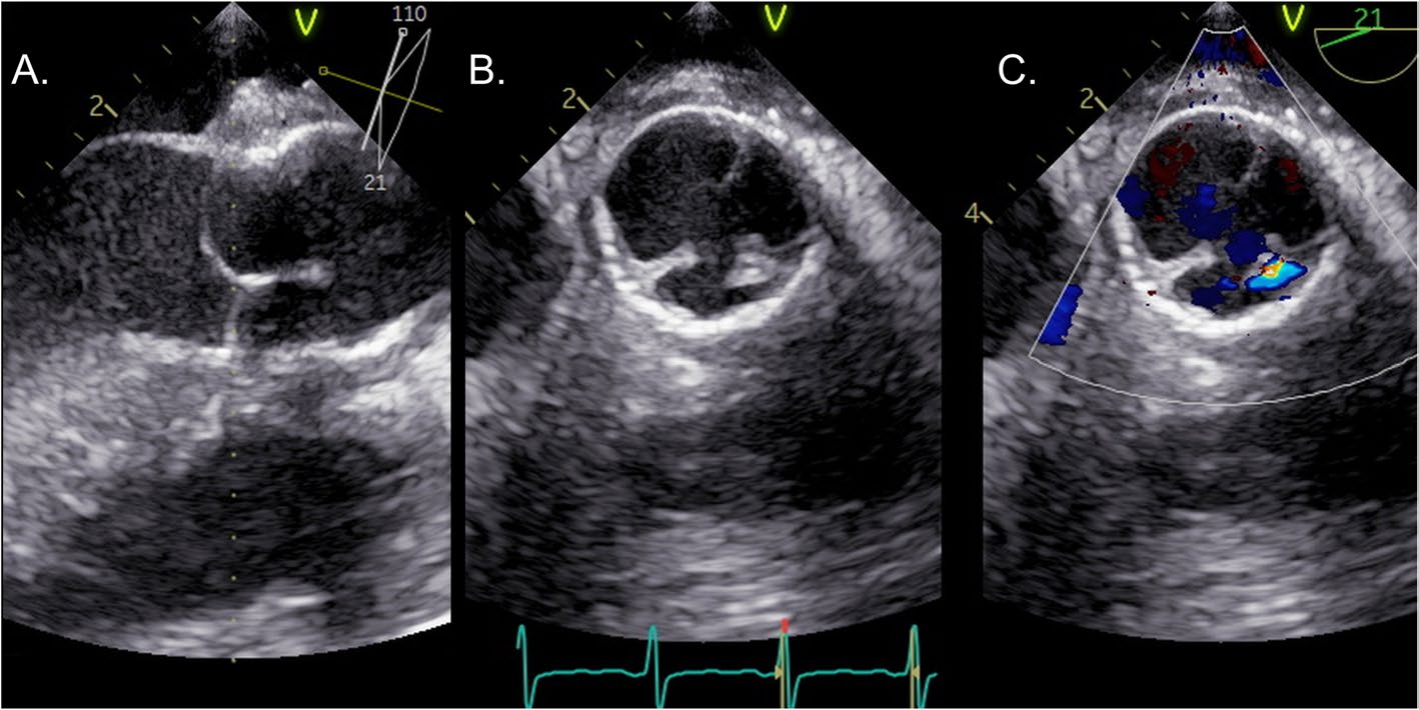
Postoperative transesophageal echocardiography. A Long-axis imaging during the diastolic phase. The coaptation of each coronary cusp appears remarkably improved. B, C Short-axis imaging during the diastolic phase. B B-mode imaging and C Color Doppler imaging. Mild regurgitation has occurred at the neo-commissure. The vena contracta is 1.0 mm
